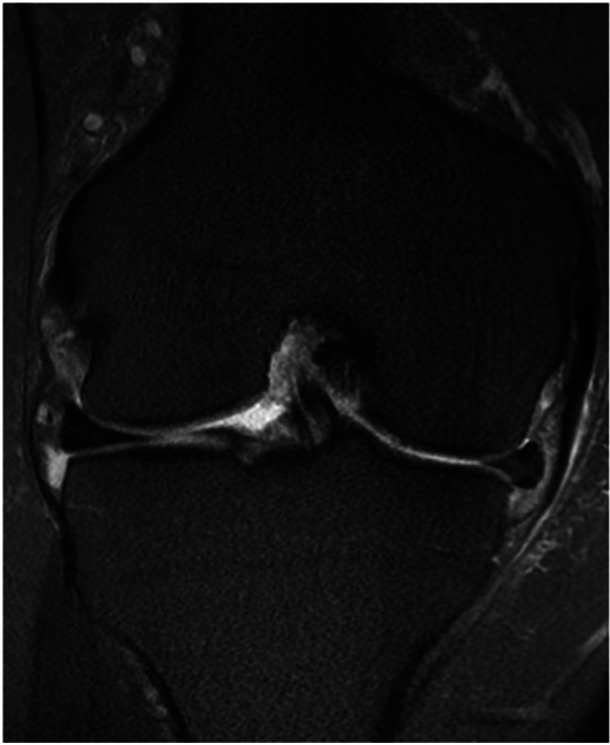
MRI coronal view of a right knee. Patient with valgus instability due to a medial collateral ligament grade 3 lesion 6 months before. In the MRI are visible both the scarred medial collateral ligament at the femoral insertion and the lesion of the meniscotibial ligament with concomitant extrusion. MRI, magnetic resonance imaging.
